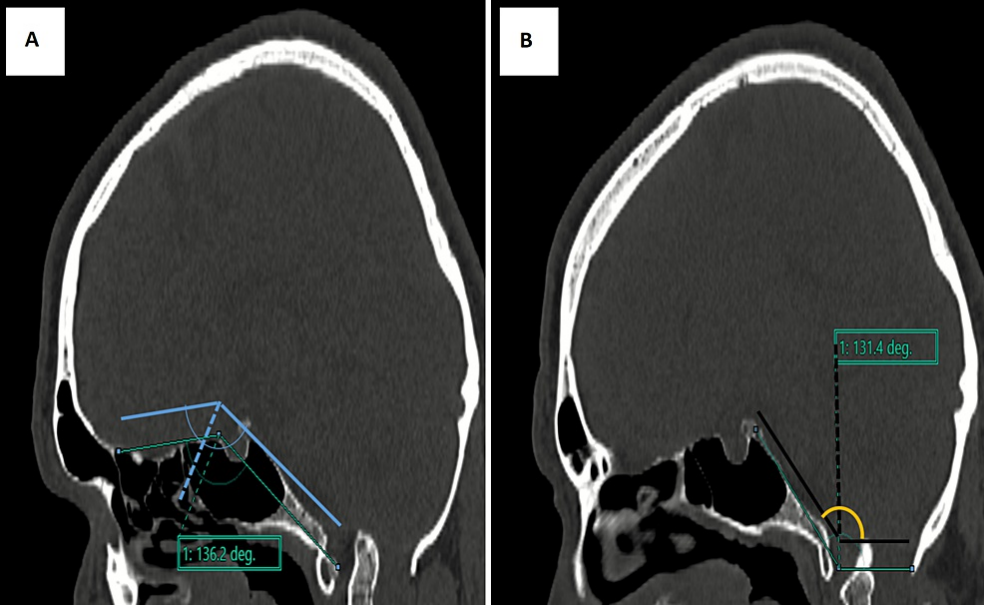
Sagittal CT images show Welcher's basal angle measuring 136.2 degrees (within normal limits) (A) and Boogard’s angle measuring 131.4 degrees (B)
